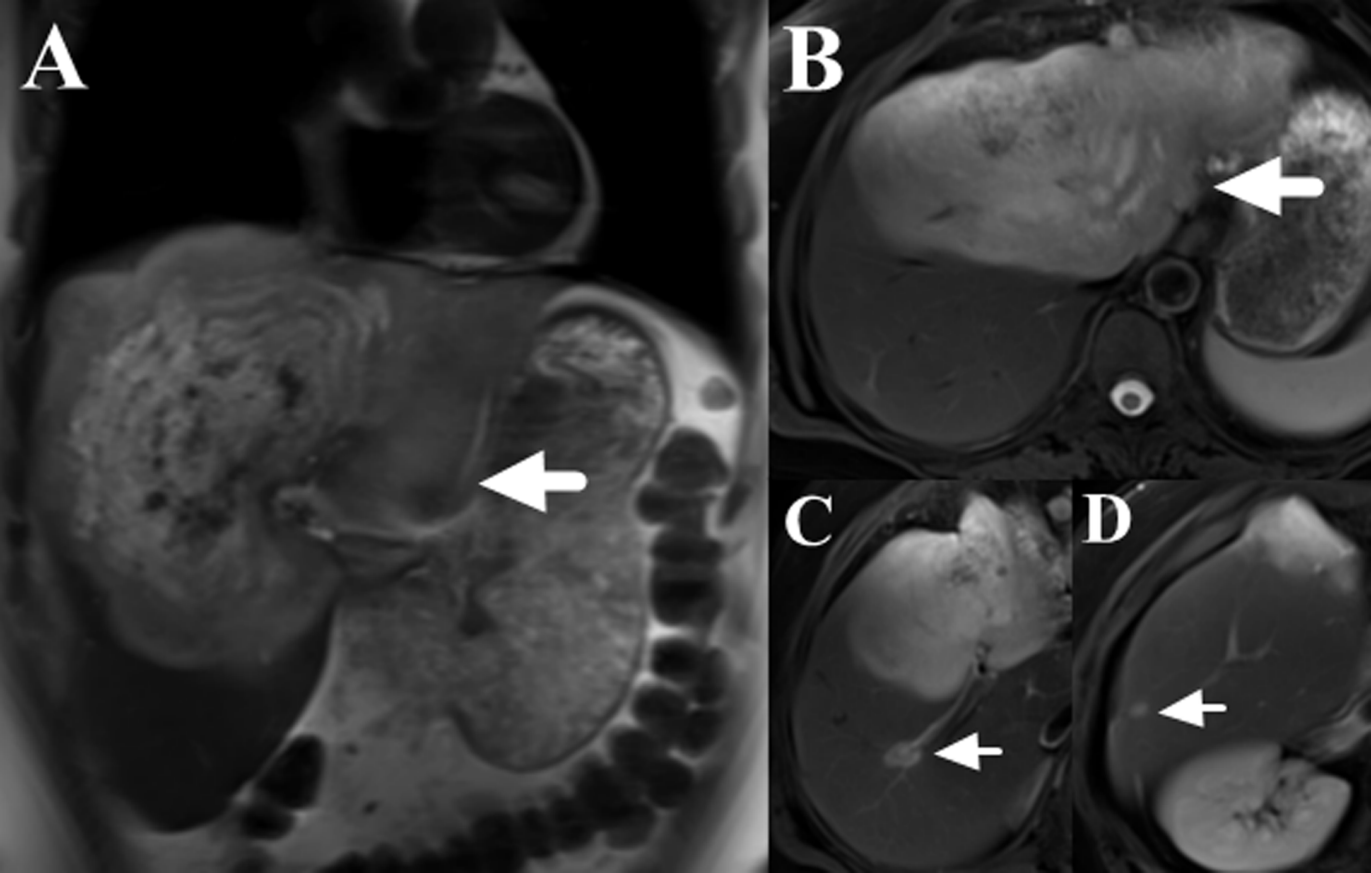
T2-weighted images of liver MRI (TR/TE: 7059/85 ms; field of view: 44 × 40 cm; matrix: 320 × 224; thickness: 8 mm), which showed a giant heterogenous high signal intensity tumor mainly located in the left lobe (A, B, marked with arrows) and multiple small high signal intensity lesions in the right lobe (C, D, marked with arrows).
